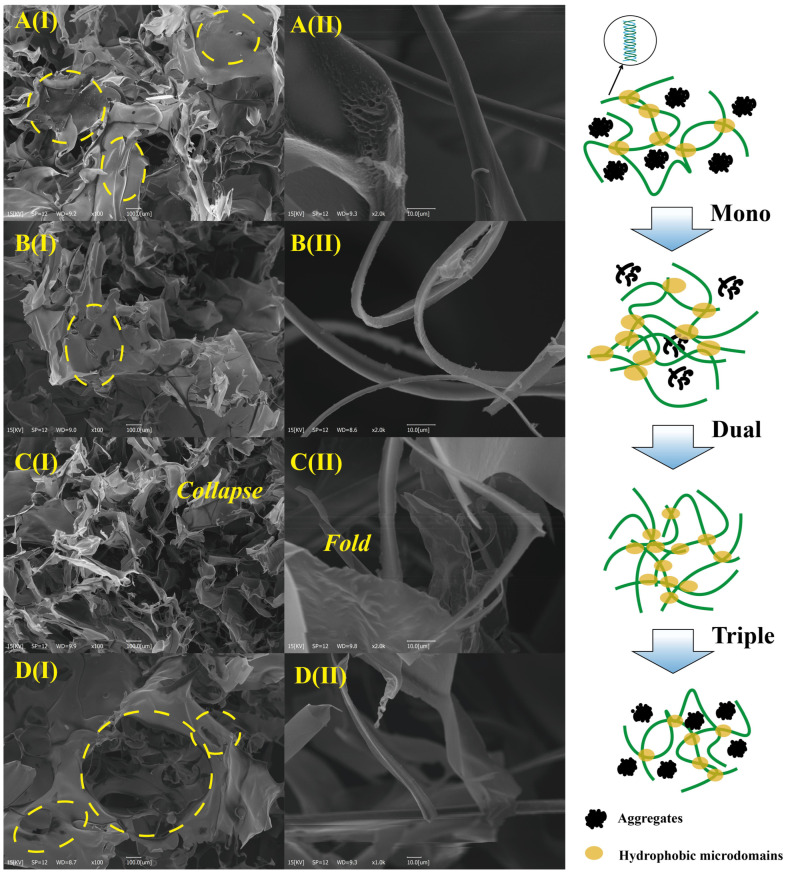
Microstructural morphologies of 4.5 g·L^−1^ XG treated by arc-shaped flat-plate ultrasonic frequency modes. (A) Untreated, (B) 33 kHz, (C) 20–40 kHz, (D) 20–50–68 kHz.
